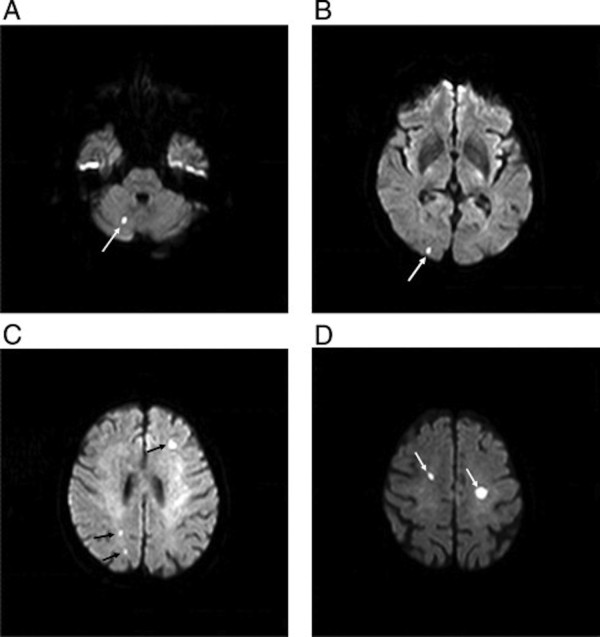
Diffusion-weighted magnetic resonance imaging (DW MRI) following transfemoral transcatheter aortic valve implantation in an 86-year-old patient. Multiple acute ischemic lesions in the right cerebellum ((A), white arrow), white occipital territory ((B), white arrow), left frontal and right parietal territories ((C), black arrows), and left and right frontal superior territories ((D), white arrows). Adapted with permission from Rodes-Cabau *et al. J Am Coll Cardiol* 2011, 57:18–28 [36].
